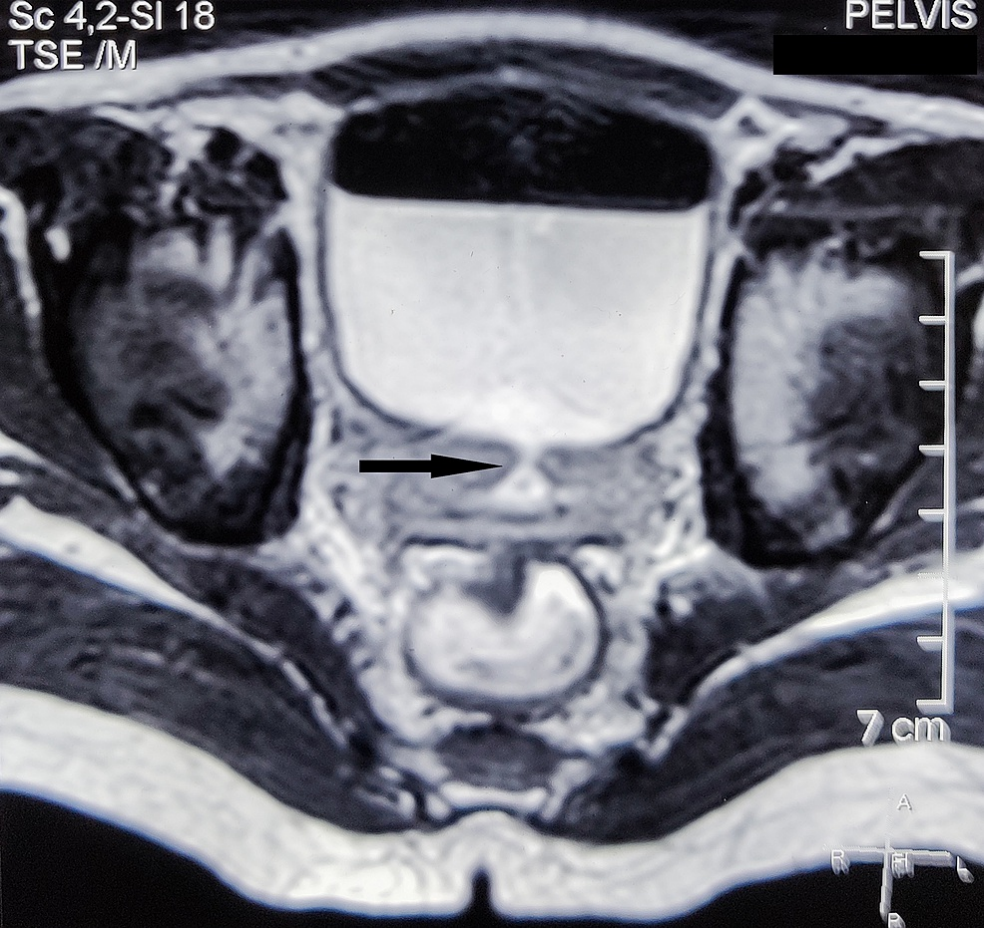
Axial section of MRI of the pelvis showing the fistulous tract (arrow)
